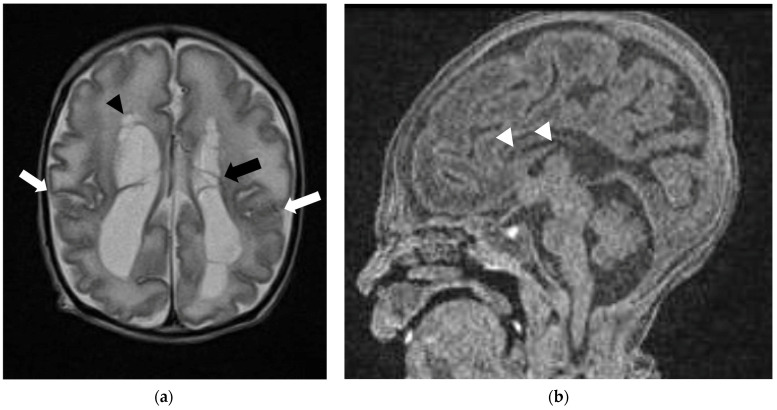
Neonate (38th week of gestation) presented with encephalopathy, subsequently confirmed PDCD with PDHA1 mutation. Antenatal ultrasound showed parenchymal cystic lesions, fetal MRI was not performed. Postnatal MRI performed on day 6 of life demonstrated findings characteristic of PDCD. (a) Axial T2W sequence shows germinolytic cysts (black arrow), periventricular cysts (black arrowhead), and gyration abnormality (white arrows); (b) sagittal T1W sequence at midline demonstrated short corpus callosum with absent posterior body, splenium, and rostrum, in keeping with callosal dysgenesis (white arrowheads).
